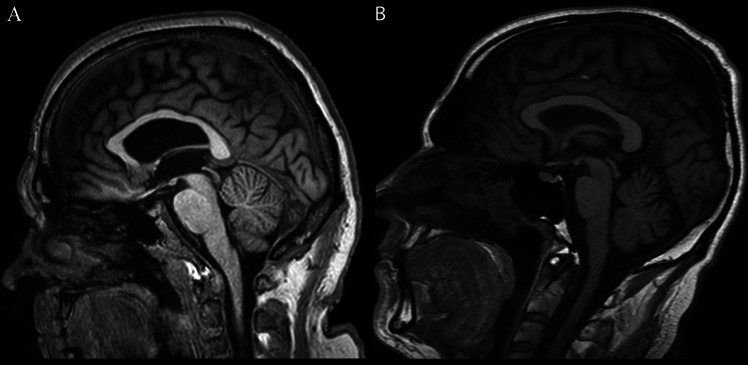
A—brain MRI (T1 W sagittal) of a healthy man at the age of 64 y, B—brain MRI (T1 W sagittal) of the Polish patient with GAA repeat expansions in FGF14 at the age of 64 y. Slight atrophy of the cerebellum is observed (the primary, secondary, and prepyramidal fissures are open, and a more prominent quadrigeminal cistern is also present)
